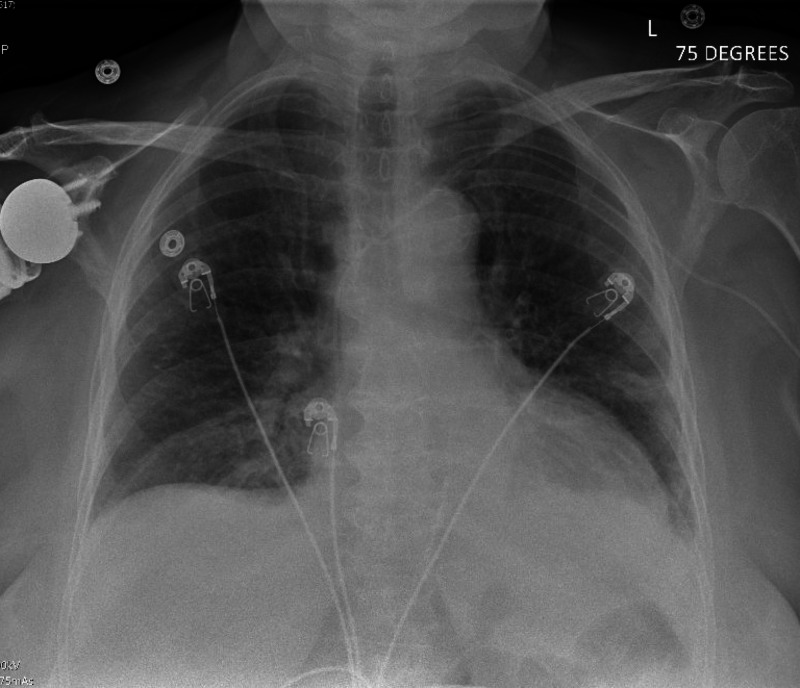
Chest X-ray showing pulmonary vascular congestion and bilateral small pleural effusions
